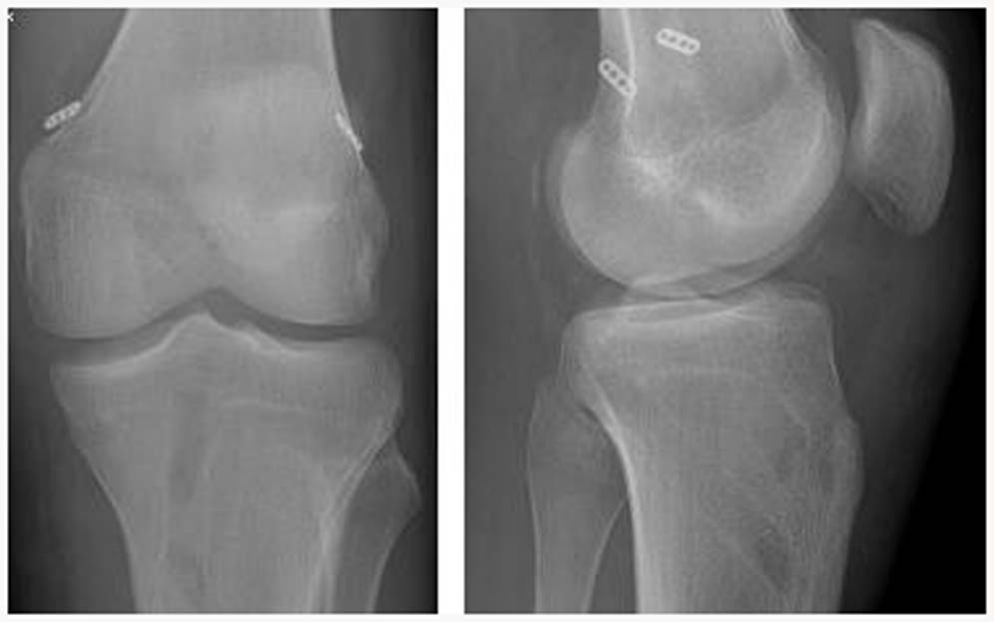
Radiographs after simultaneous ACL and PCL reconstruction.
